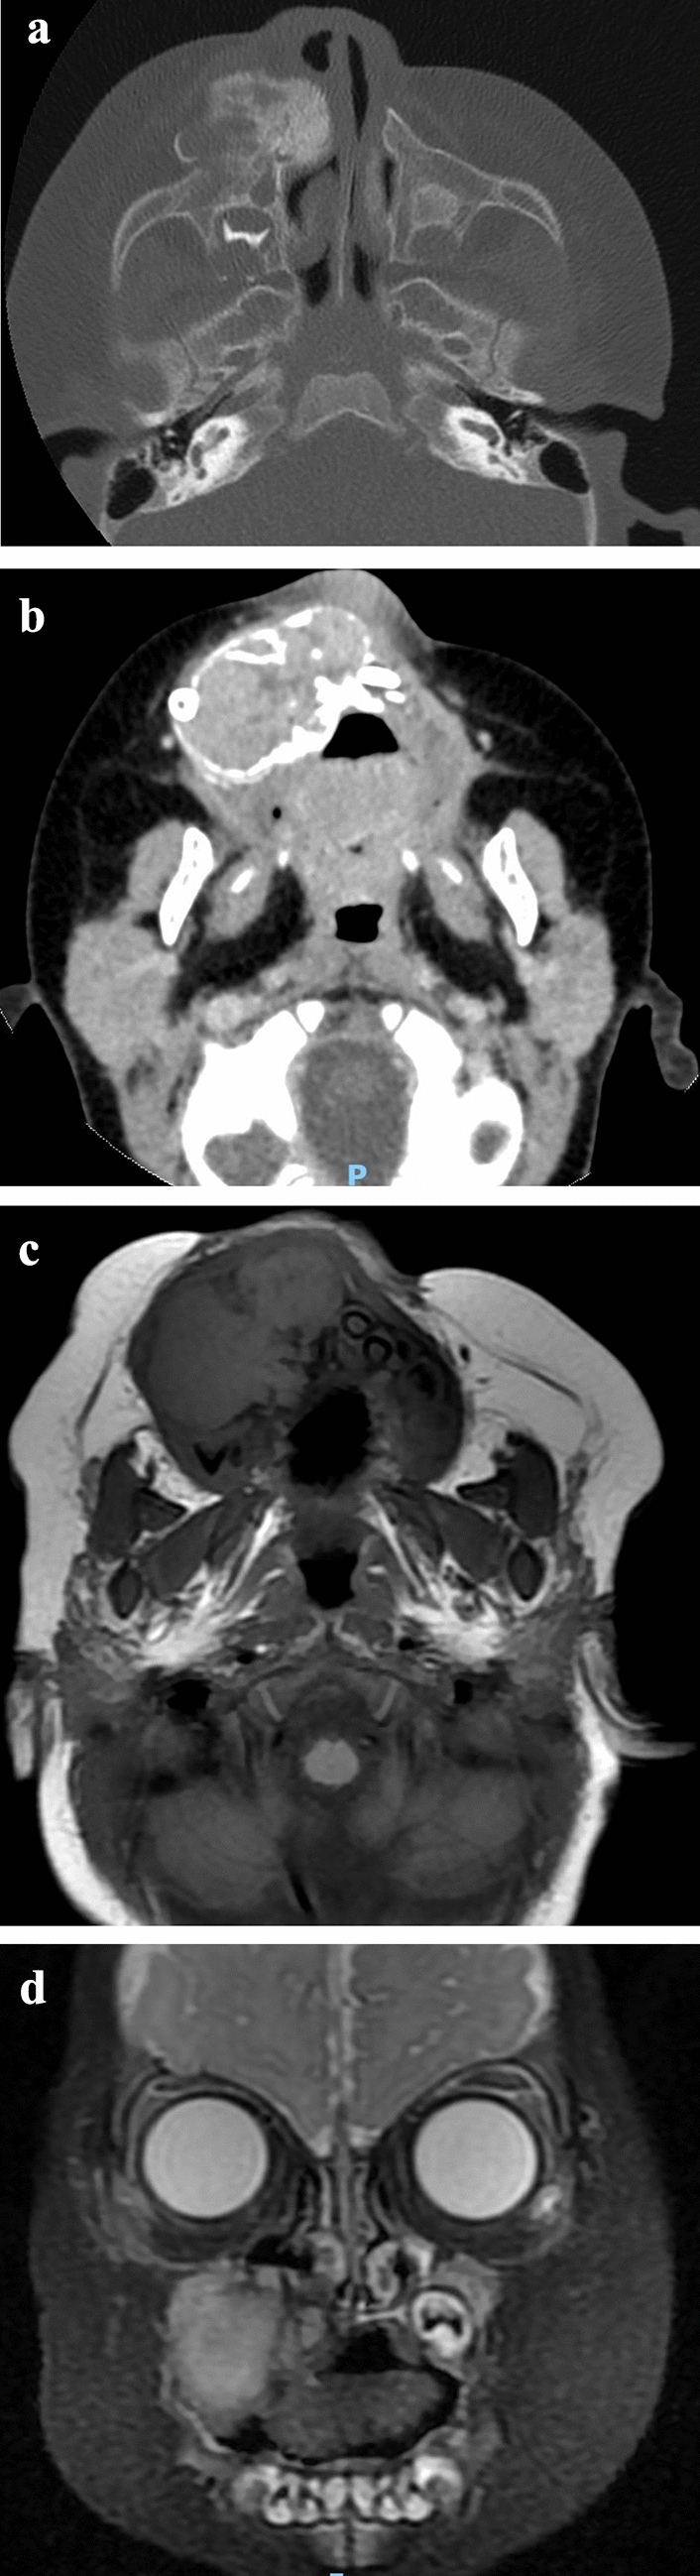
Case 4. a/b axial CT images showed irregular bone destruction and cortical bone expansion, with no signs of periosteal reaction. Teeth in the lesion were displaced. c axial T1 weighted image showed isointense or mild hyper-intense signal. d coronal T2 weighted also showed isointense or mild hyper-intense signal
